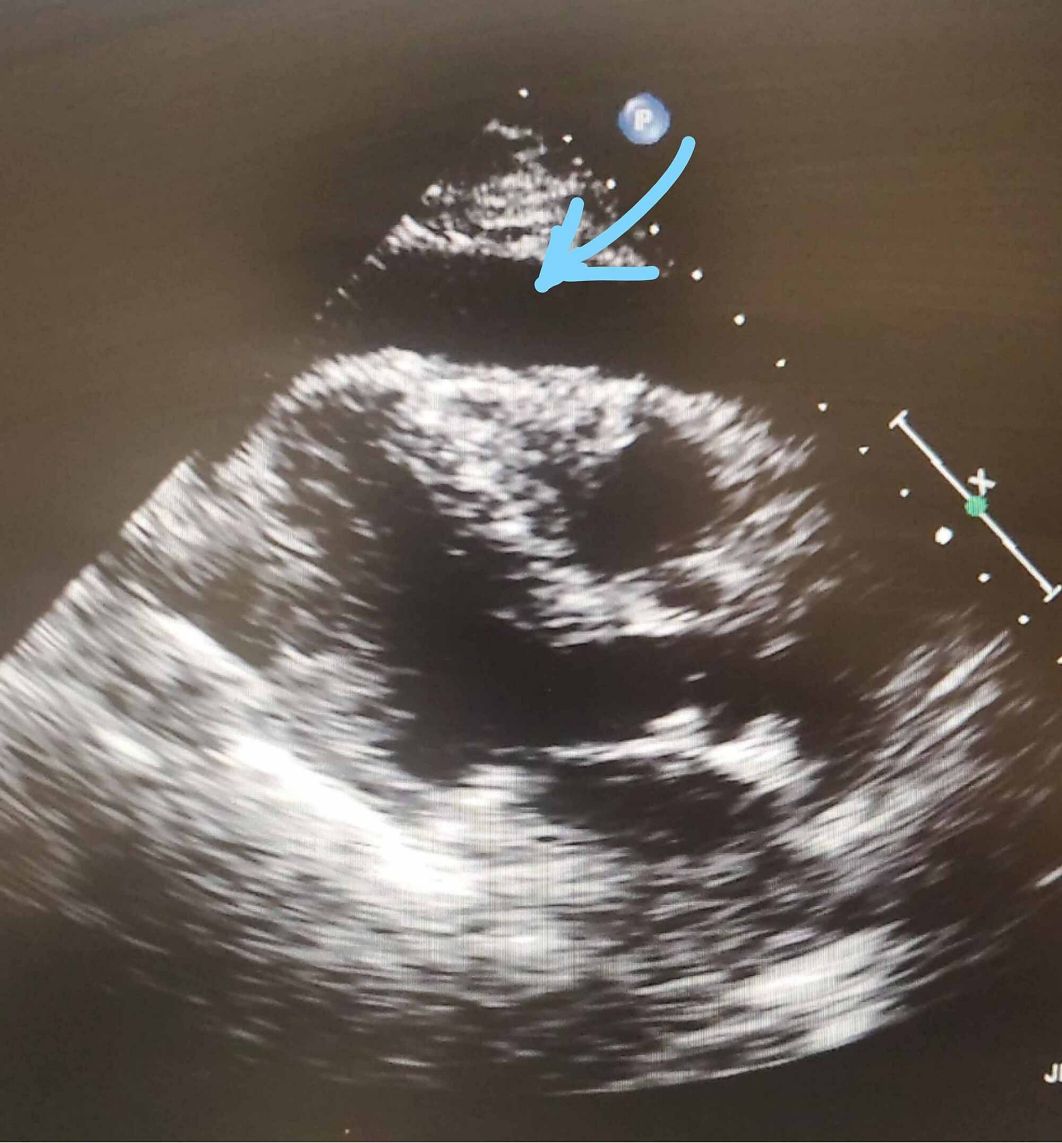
Echo showing parasternal long axis view. Pericardial effusion (blue arrow)
